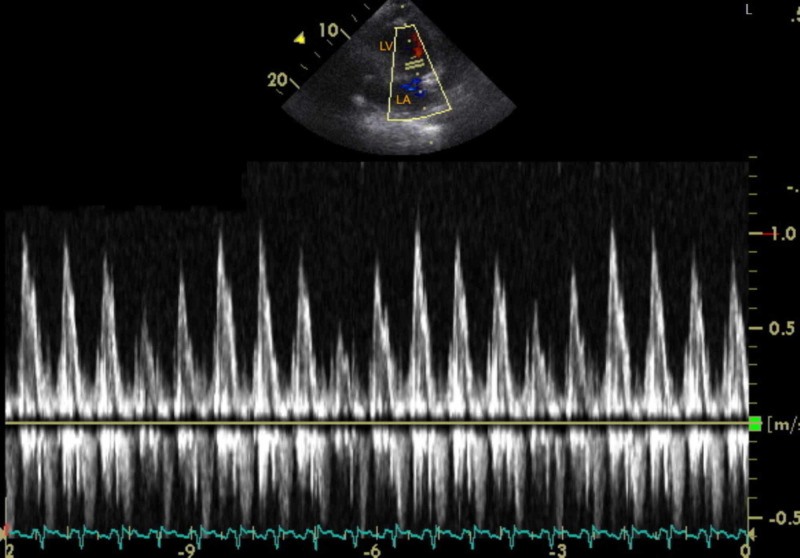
Transthoracic echocardiogram with pulse wave doppler The image shows mitral inflow velocity on pulse wave doppler showing about 40% respiratory-driven variation LA: left atrium; LV: left ventricle
